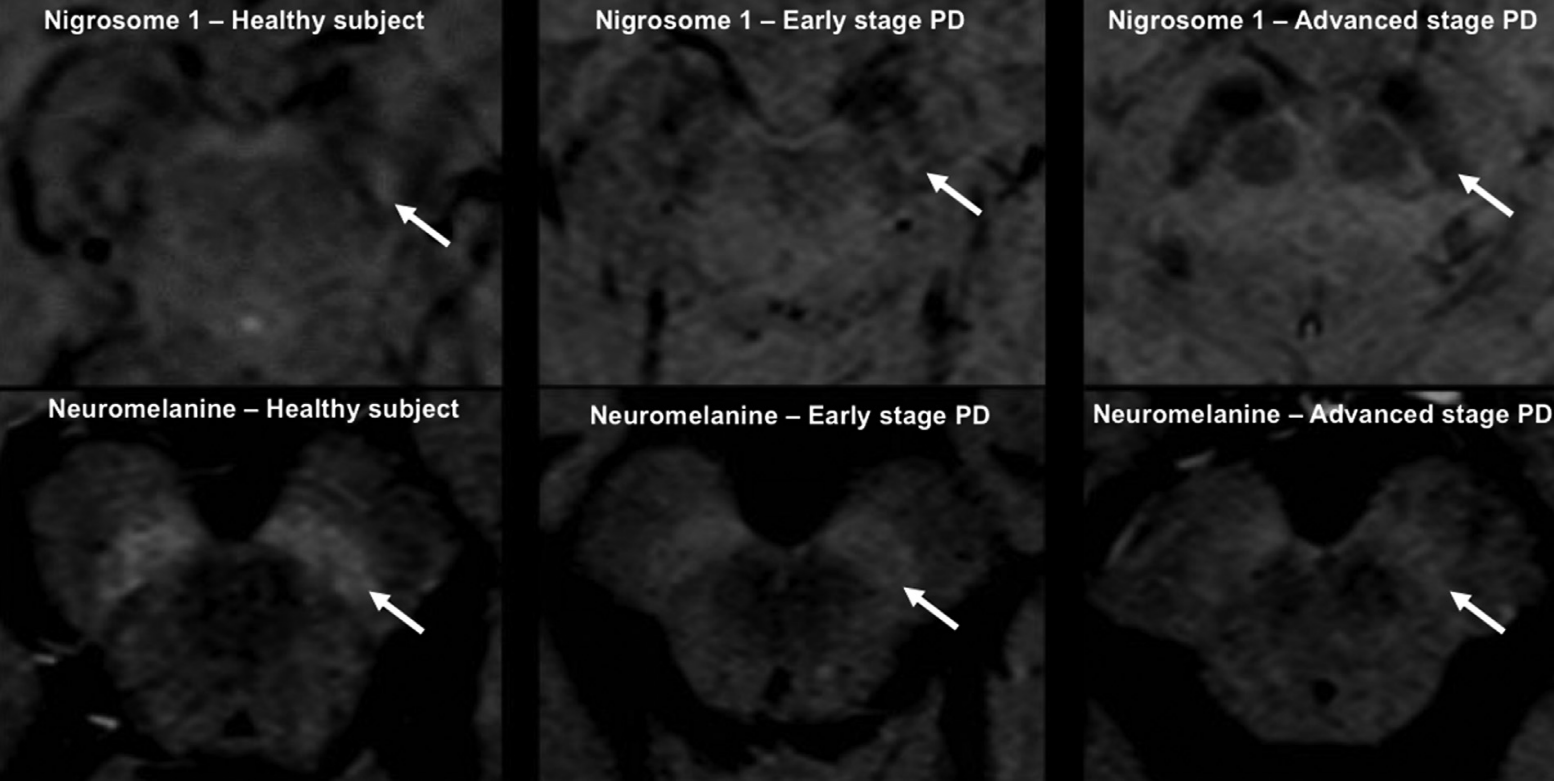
Brain MRI with nigrosome and neuromelanin findings, respectively in healthy subjects, early stage of PD and advanced stage of PD. In healthy subjects there is a clear swallow tail appearance in nigrosome imaging and hyperintense signal in the substantia nigra in the neuromelanine sensitive MRI. On the other hand, in advanced stages of PD, there is absence of the swallow tail appearance in nigrosome imaging and decrease of the hyperintensity in neuromelanine imaging. Early stage of PD presents with intermediate findings between both conditions described above. This image was kindly supplied by Dr. Victor Hugo Rocha Marussi, from Beneficência Portuguesa, São Paulo, Brazil.
